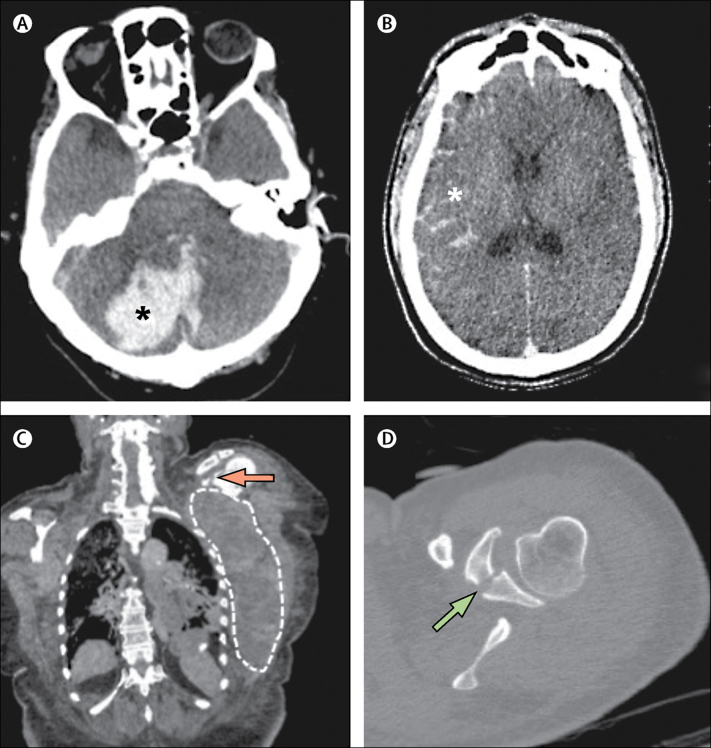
Images from post-mortem CT with targeted coronary angiography in three cases of haemorrhage (A) Axial brain image in a 73-year-old woman who collapsed and then had a cardiac arrest after a short interval. The autopsy report described a small amount of subarachnoid blood and normal cerebral cortex, but did not describe the cerebellum, whereas post-mortem CT with targeted coronary angiography (PMCTA) showed a clear clinically significant cerebellar haemorrhage, as indicated by an asterisk. Autopsy gave coronary artery disease as the cause of death, which, although also detected by PMCTA, was clearly incorrect. (B) Axial brain image of a 33-year-old man with type 1 diabetes and alcohol addiction. Toxicology showed evidence of clinically significant diabetic ketoacidosis, and both PMCTA and autopsy give the primary cause of death as diabetic ketoacidosis, but autopsy failed to report the clear subarachnoid haemorrhage, as indicated by an asterisk, which although not extensive enough to definitely cause death, might have substantially contributed to death. (C, D) An 84-year-old woman who was taking anticoagulation treatment with documented declining haemoglobin concentrations in the days leading to her death. She died from myocardial insufficiency secondary to hypovolaemia and anaemia agreed on both autopsy and PMCTA. However, autopsy did not find a bleeding source and attributed it to gastric erosions. PMCTA clearly showed a left scapula fracture (arrow) with approximately 1 L of blood in the left chest wall (dashed line).
